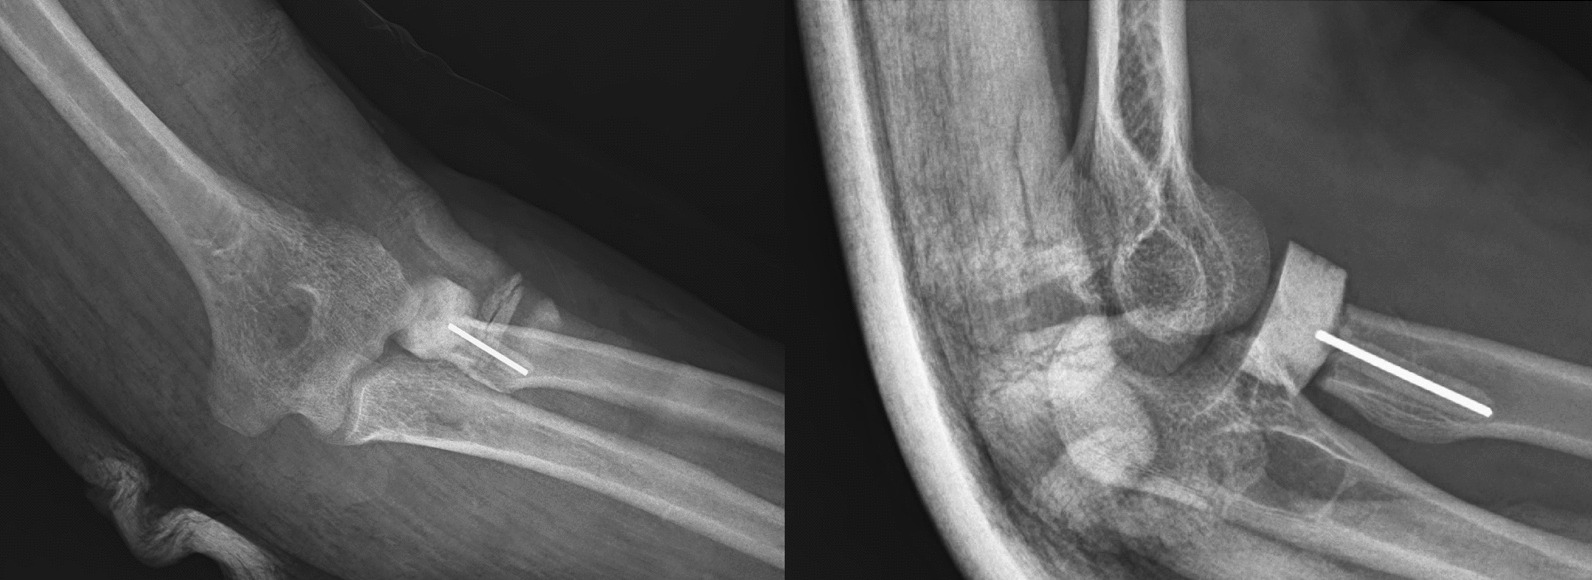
Postoperative left elbow X-rays
